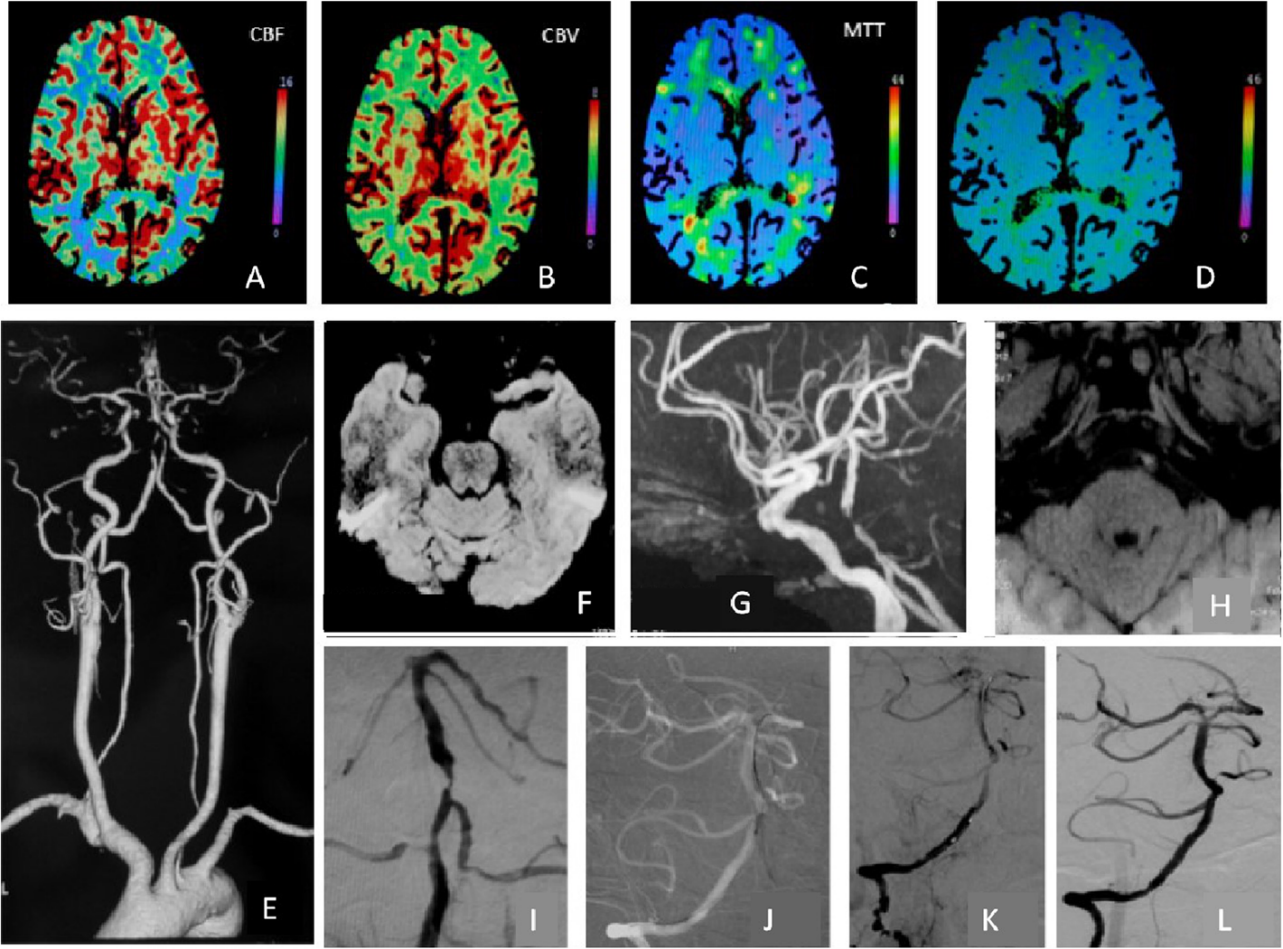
Multimodal imaging assessment and surgical procedures. a-d Preoperative, posterior circulation CTP perfusion was abnormal. e CTA of the head and neck demonstrates severe stenosis of the lower basilar artery. (-h DWI images showed no acute cerebral infarction, MRA images show severe stenosis in the lower basilar artery, HR-MRI images show basilar eccentric stenosis. i Before the operation, DSA images showed severe stenosis in the lower basilar artery. j-k During the procedure, balloon expansion and stent implantation were performed. l Postoperative, DSA images showed significant improvement in severe basilar stenosis
